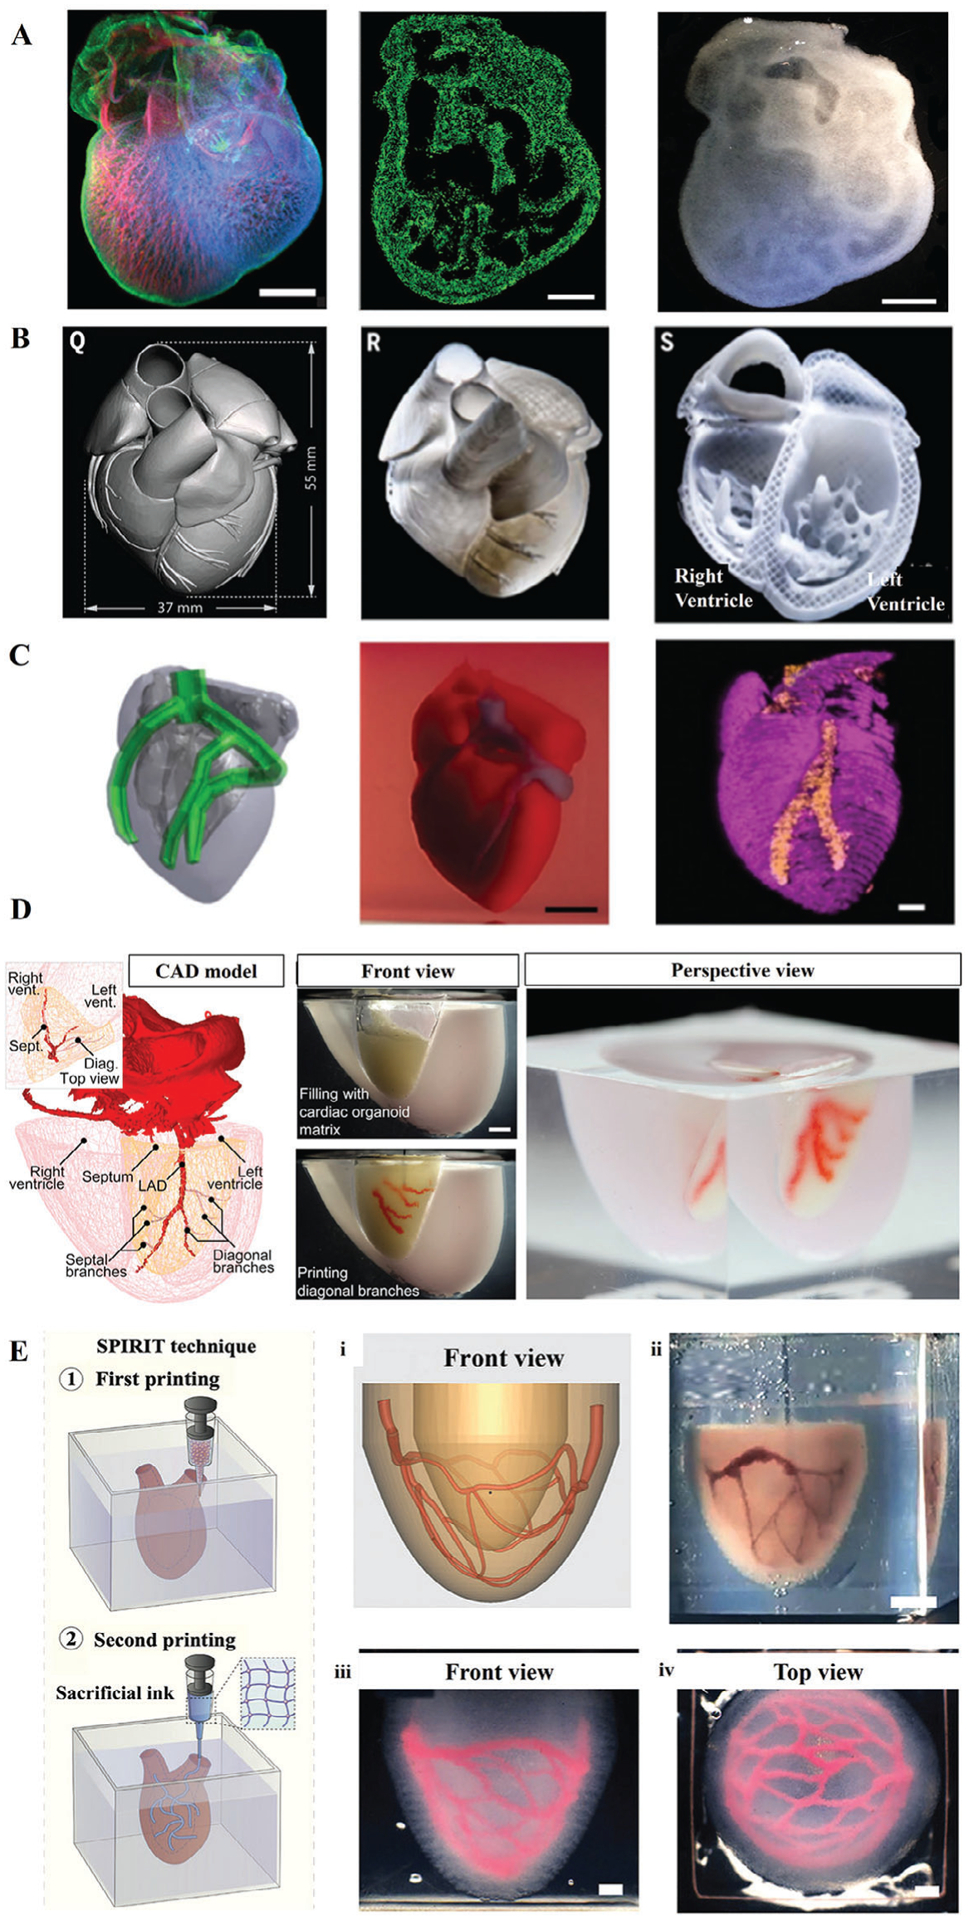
A) A 3D image of a 5-day-old embryonic chick heart (left); a cross–section image (middle) and a dark-field image (right) of the 3D printed heart; Adapated with permission.^[24]^ Copyright 2015, American Association for the Advancement of Science. B) The designed heart model (left); the side (middle) and cross–sectional view (right) of the bioprinted heart model; Adapated with permission.^[18]^ Copyright 2019, American Association for the Advancement of Science. C) Images of the magnetic resonance imaging (MRI)-derived 3D human heart (left), FRESH-printed heart (middle), and cross–sectional view of the heart (right); Adapated with permission.^[210]^ Copyright 2019, Wiley-VCH. D) A 3D model of a normal human heart and vascular branches that were bioprinted into a mode of septal-anterior wall wedge; Adapated with permission.^[23]^ Copyright 2019, American Association for the Advancement of Science. E) Perfusable ventricle constructs fabricated by SPIRIT: (i) Front view of a 3D model of the hierarchical vascular network within a ventricle construct. (ii) Extruded gelatin ink into the bioprinted ventricle. (iii–iv) The optical images showing a 3D-printed ventricle with a densely packed vascular network (indicated by red dye) at the front view (iii) and top view (iv); Adapated with permission.^[20]^ Copyright 2023, Wiley-VCH.
